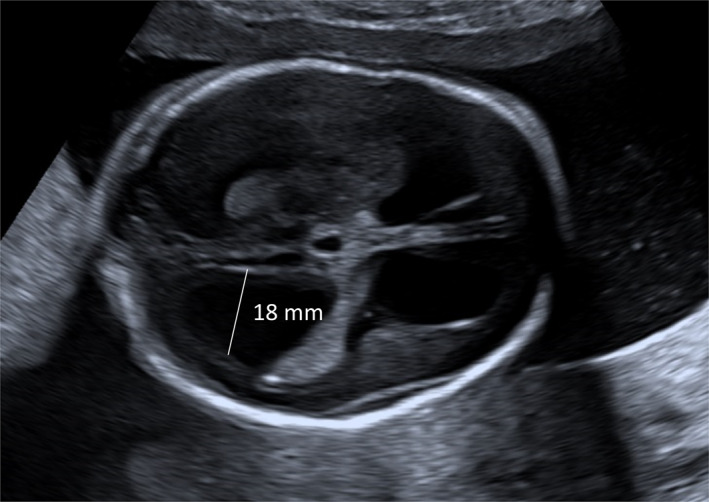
Ultrasound at 19 weeks of gestation, showing severe ventriculomegaly.
